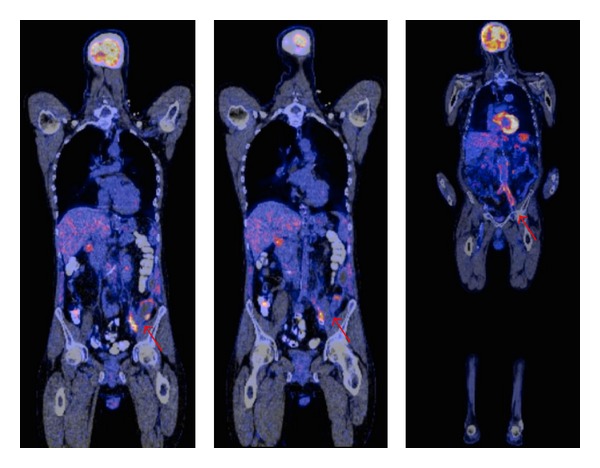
Coronal view of fused ^18^F-FDG PET/CT image of a culture proven not infected vascular prosthetic graft. In this particular case, a 45-year-old male patient underwent emergency surgery by placement of a Viabahn in the left common iliac artery because of rupture. He was admitted to the hospital 6 weeks later with fever and sepsis. ^18^F-FDG PET/CT scanning showed increased FDG uptake at the level of the left iliac artery. Ultimately, no bacteria were cultured from the graft under antibiotic therapy.
